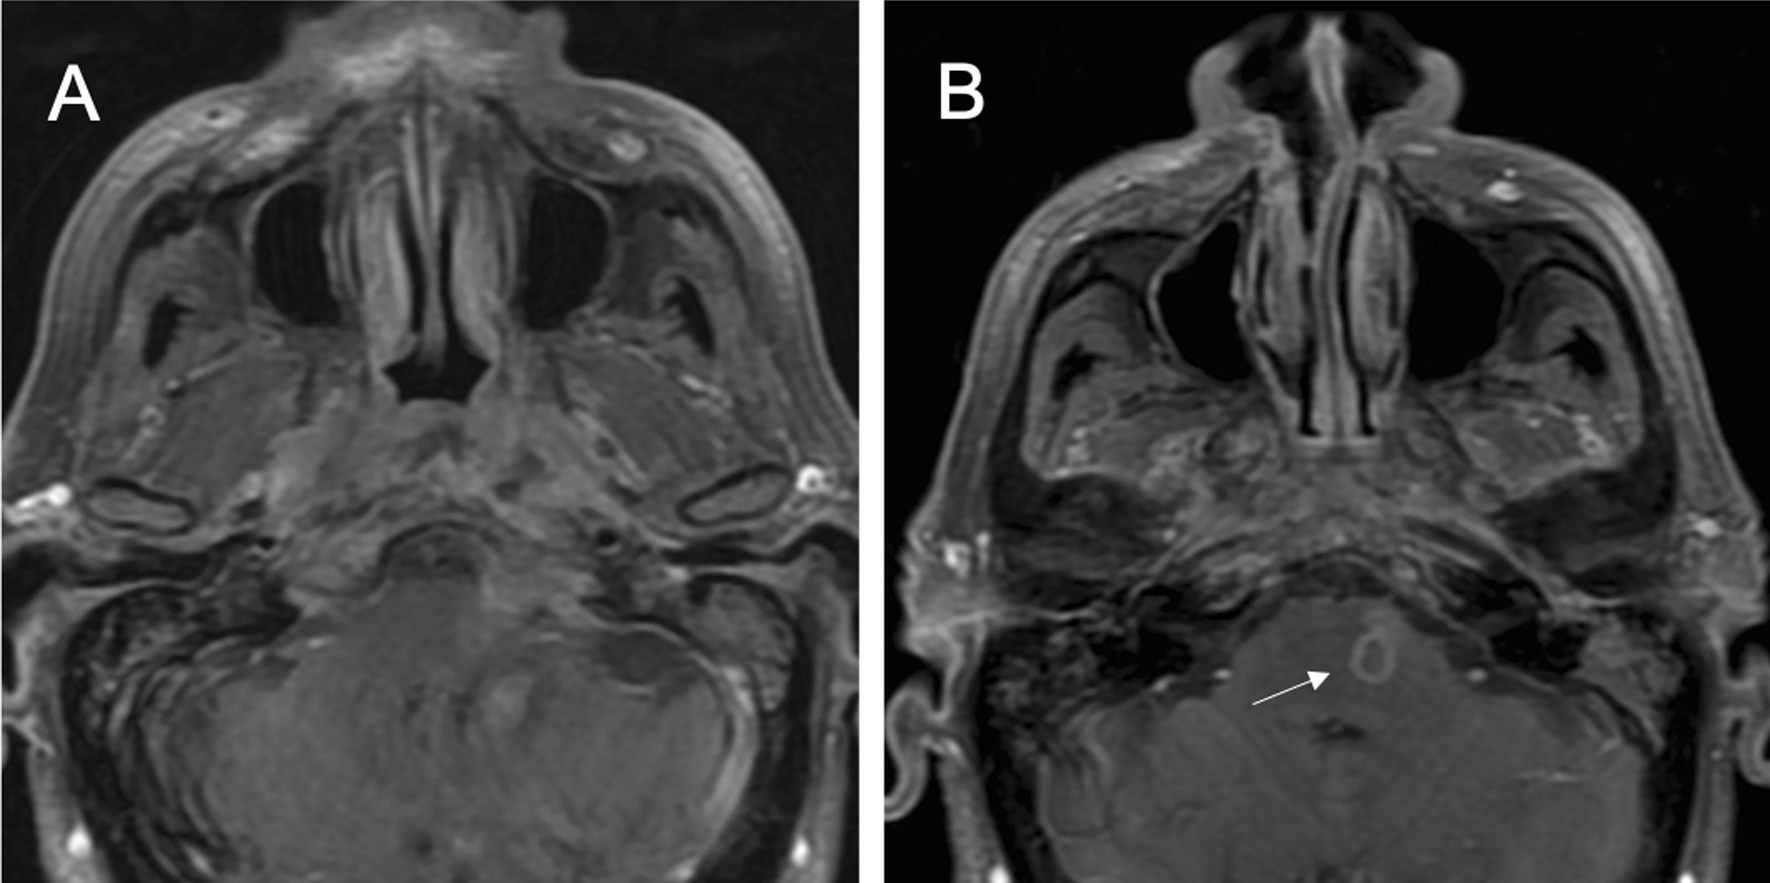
A second MR study performed one month later showed persistence of the lesion previously observed in the skull base. Extradural extension of the lesion (a) and an intra-axial lesion located on the left side of the pons with peripheral enhancement (b, *white arrow*) were seen on post- contrast T1-weighted images
